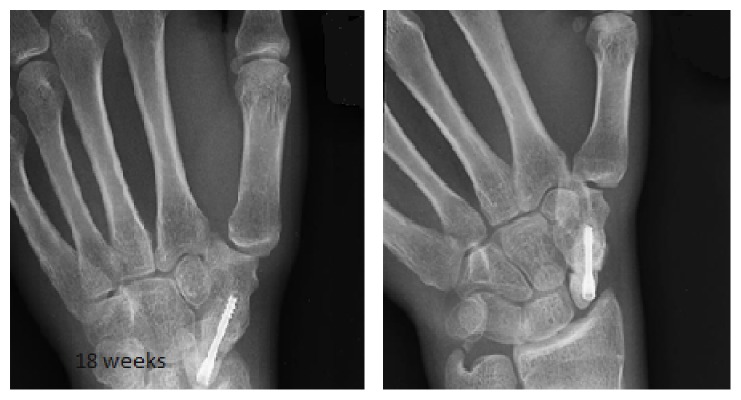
Radiographs show fracture fixation 18 weeks postoperatively.
